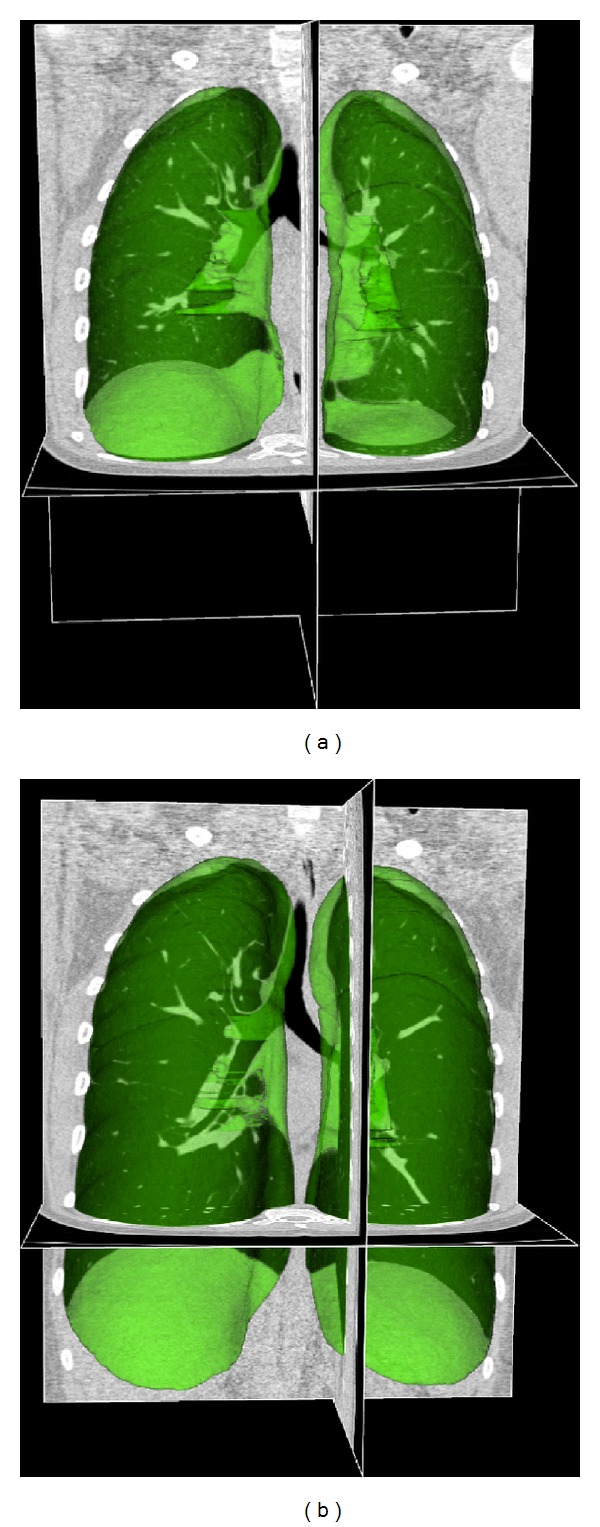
Pulmonary CT images acquired at breath hold (a) maximum exhale and (b) maximum inhale with renderings of the lung segmentations (green objects).
